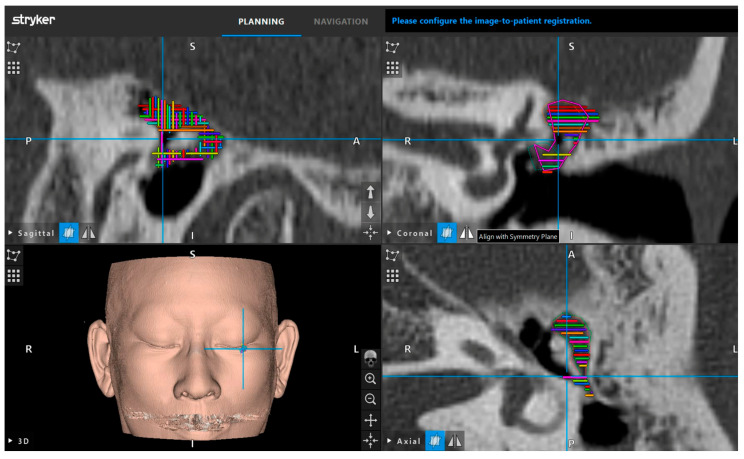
Navigation monitor after completion of tracing of lesion. The lesion was traced every 1.2 mm in three computed tomography (CT) directions and was ready for the augmented reality (AR) display after pre-planning was performed. The upper-left, upper-right, and lower-right images are sagittal, coronal, and axial slices, respectively. The tracing colors are freely selectable, and a variety of colors were used in this case.
